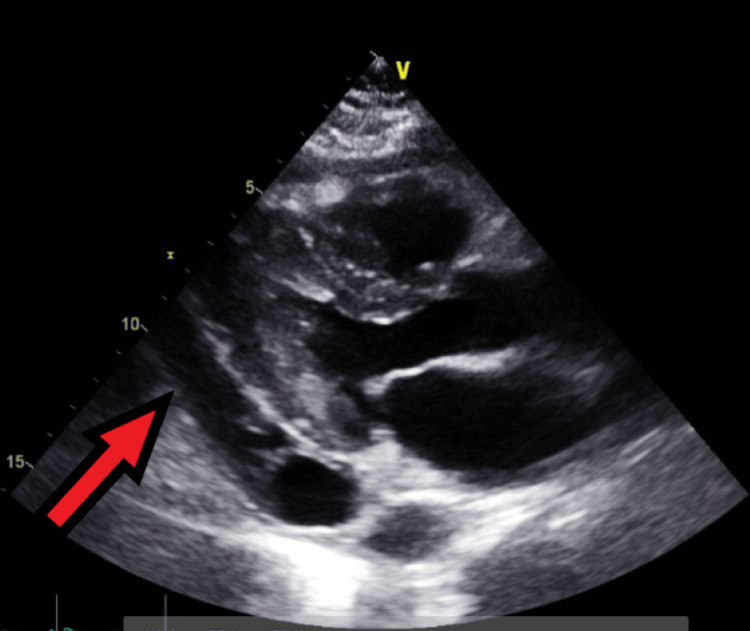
Follow-up transthoracic echocardiography on the next day after admission - image 1 Transthoracic echocardiography on the next day; parasternal long-axis view shows increased pericardial fluid collection (red arrow), indicative of large pericardial effusion
